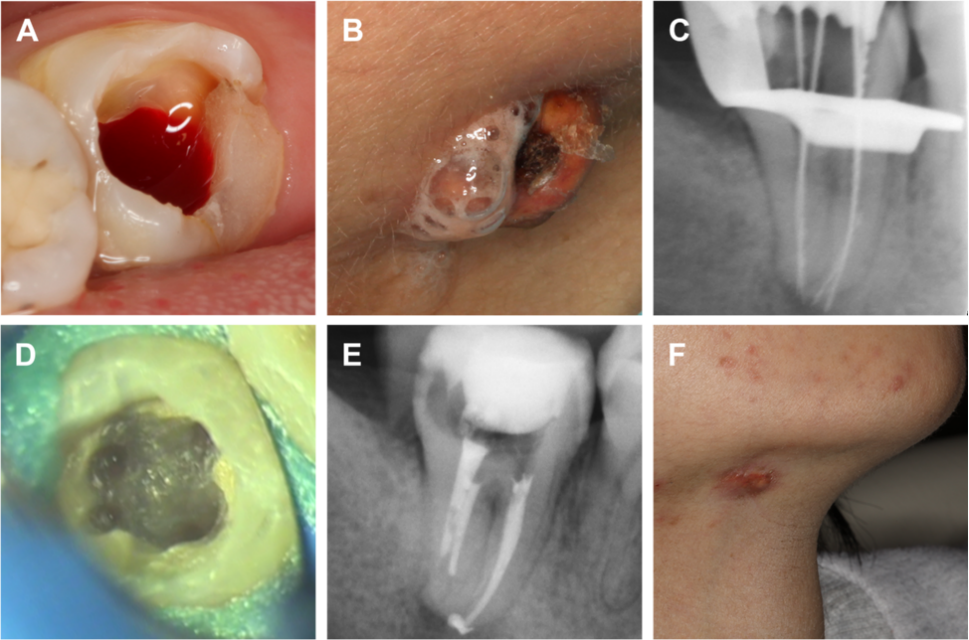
Clinical and radiographic records during the treatment. (A) Plentiful errhysis originating from the pulp chamber during and after accessing the cavity. (B) 3% hydrogen peroxide along with bloody pus leaking from the extra-oral drainage sinus during irrigation. (C) Working length determination. (D) Floor of the pulp chamber showing four orifices after preparation. (E) The root canals were packed with Vitapex after preparation. (F) The resolution of the sinus tract after dressing for one month.
